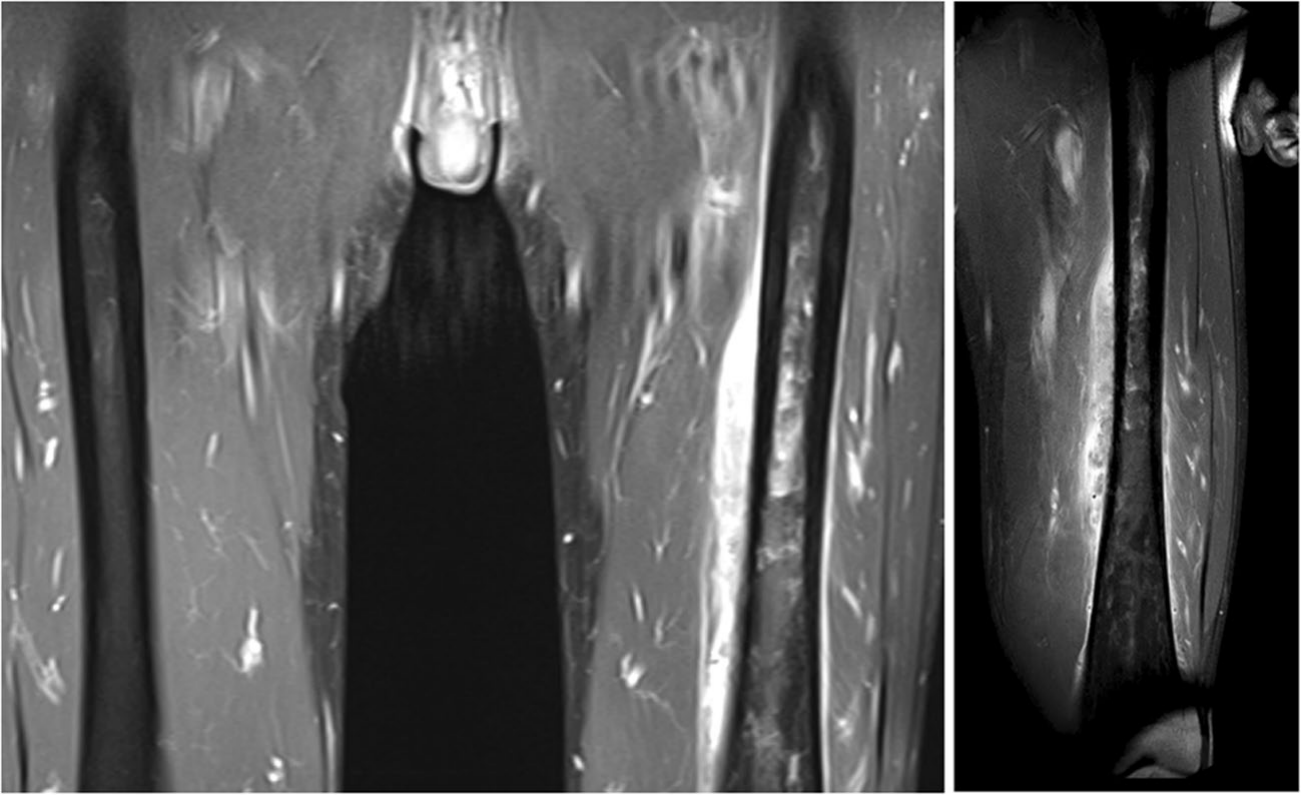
MRI scan (number 2) of both femurs (left) and a close-up of the left femur (right) showing a parosteal lesion, oedema, and enhancement of the musculus vastus intermedius (28 × 11 × 140 mm) including general oedema in the surrounding musculature and permeative process of the left femoral diaphysis
